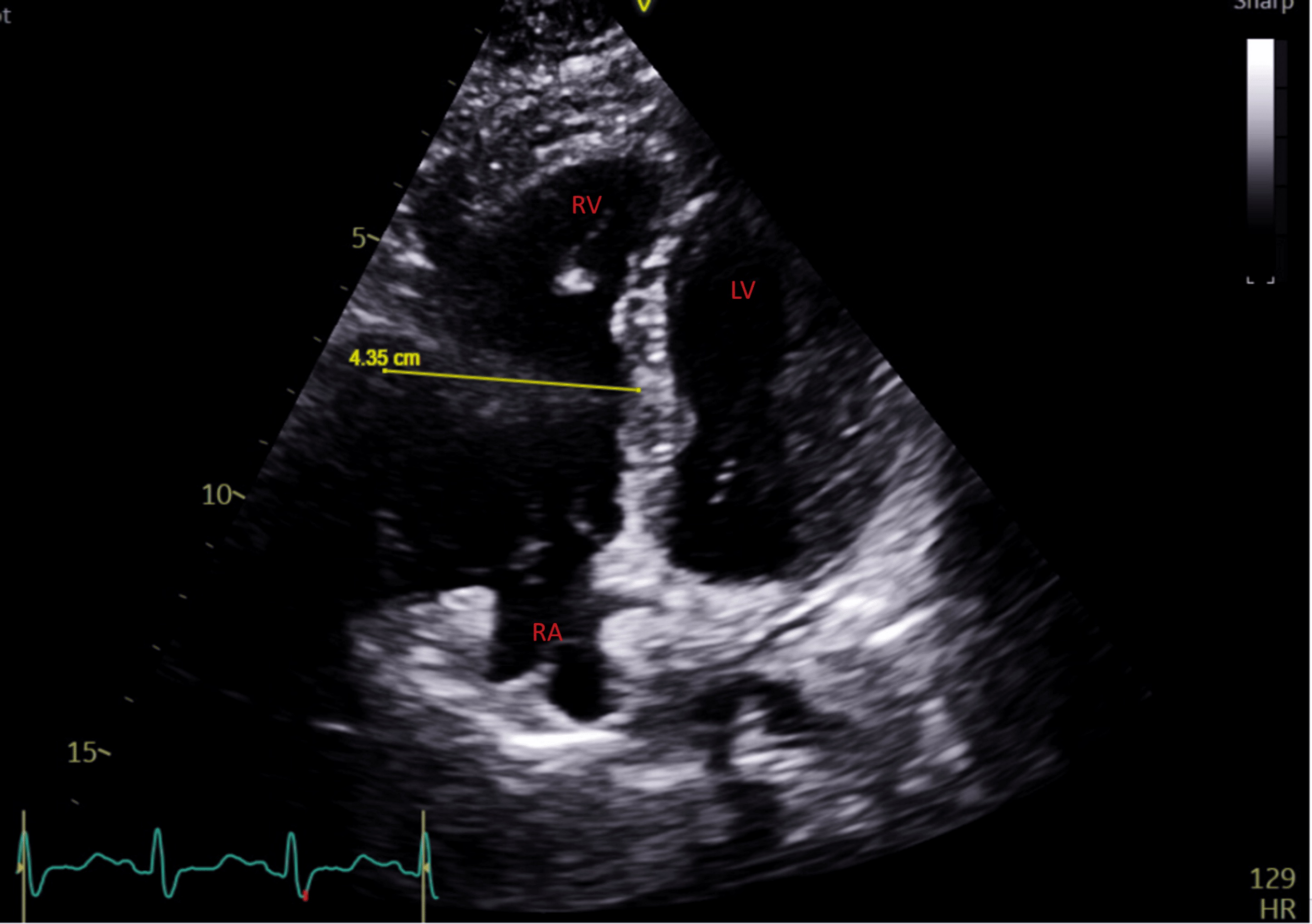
Significant right ventricular dilatation on echocardiography. The mid-diameter of the right ventricle on echocardiography measured 4.35 cm, which is above the cutoff value of 3.5 cm.
